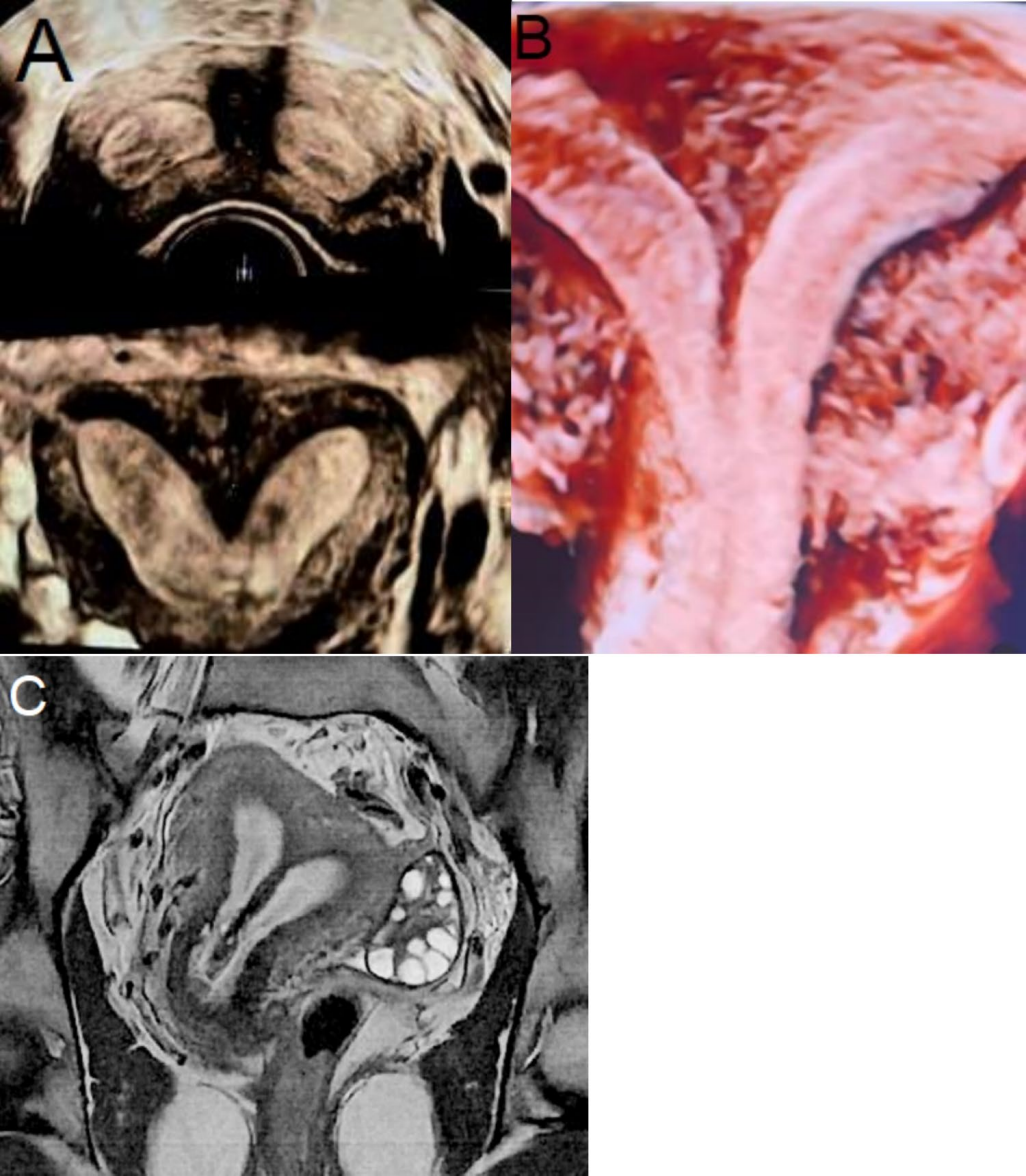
Imaging tools. A Transvaginal ultrasound (subseptus), B Transvaginal ultrasound (subseptus), C- Magnetic resonance imaging (septus)
